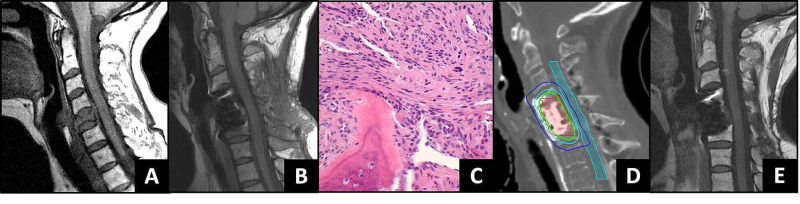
Spine Oligometastatic Treatment Twenty-six years after her initial diagnosis of a grade 1 intracranial meningioma and eight years following intracranial recurrence as a grade II, she presented with symptomatic C5 metastasis (panel A – sagittal T1 pre-contrast MRI with complete replacement of the vertebral body with pathologic fracture. There was a para-spinous extension into the soft tissue of the neck along the vertebral arteries). Following subtotal resection and stabilization (panel B – post-surgical T1 pre-contrast MRI), pathology revealed a grade II meningioma within the bone (panel C), concordant with the prior intracranial recurrence (in Figure 1B). She had definitive postoperative spinal stereotactic radiosurgery (SRS) to her residual tumor (panel D – spinal SRS plan. 27 Gy in three fractions (green isodose line) targeted the postoperative resection site (red contour)). The spinal cord (blue contour) was limited to a maximum of 21 Gy (cyan isodose line). Three years following spine SRS, she remains without evidence of spine (panel E), brain, or metastatic progression.
